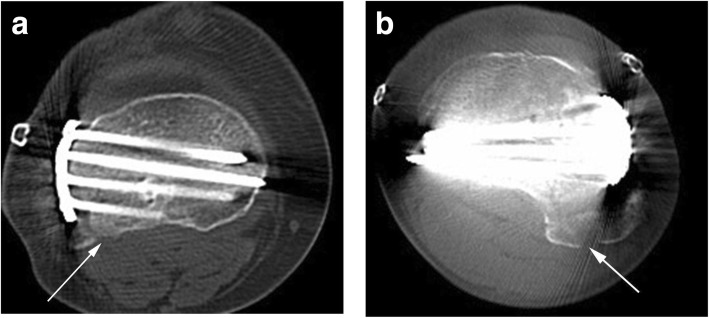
The CT scan used to evaluate the posterolateral fracture fixation. a Cross CT scan showed screws fixation of posterolateral tibial plateau fracture, the arrow indicated posterolateral fracture was completely fixed, no free bone fragment; b Cross CT scan showed only part of the posterolateral fracture was fixed, the arrow indicated one bone fragment was free
